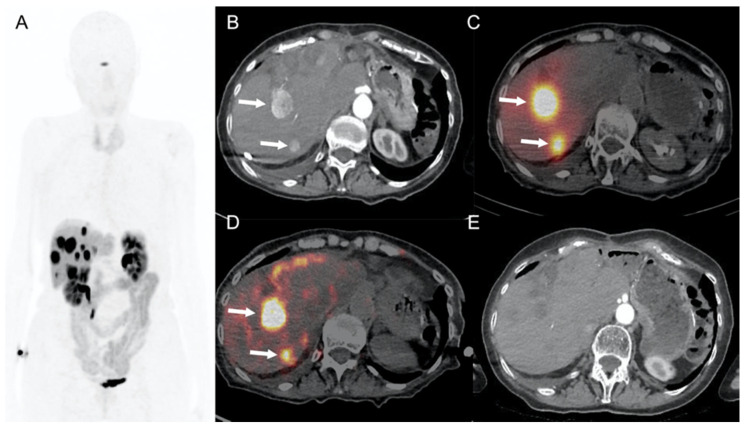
A 77-year-old woman with a previous history of surgically treated pancreatic grade 1 NEN (Ki67 = 1%) presented during follow-up with exclusive hepatic relapse. Multidisciplinary committee stated for ^90^Y-selective internal radiation therapy (SIRT) treatment. (A) Restaging anterior MIP of ^68^Ga-DOTATOC PET performed during follow-up; (B) arterial-phase contrast-enhanced CT obtained before SIRT; (C) pretreatment scintigraphy (axial SPECT/CT) performed during work-up after injection of macroaggregated albumin labeled with ^99m^Technetium showing intense uptake by metastasis (arrows) and slight uptake by normal liver parenchyma; (D) posttreatment PET/CT (axial slice) confirming the intense uptake by liver metastasis (arrows) perfectly concordant with pretreatment scintigraphy; and (E) 6-month CT (arterial phase, axial slice) after SIRT showing tumoral involvement and treatment efficacy.
